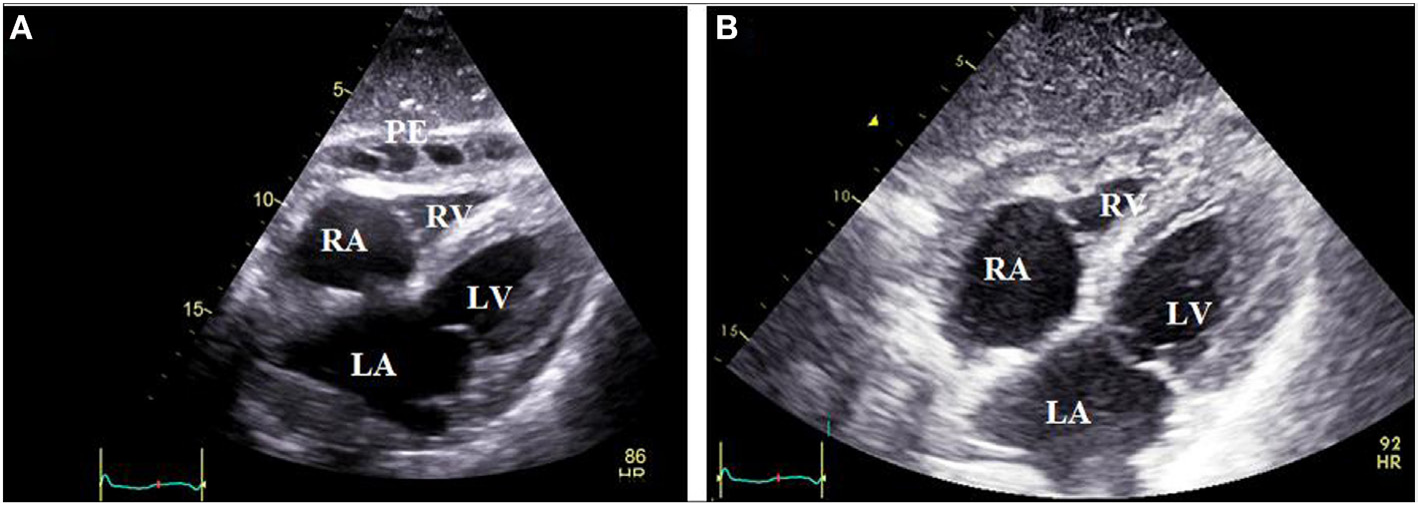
Subxiphoid echocardiographic view of a patient with colchicine-resistant steroid dependent idiopathic recurrent pericarditis. Panel (A) depicts pericardial effusion mainly in the anterior pericardial space with thick adhesions between visceral and parietal pericardium, which developed during the steroid tapering process. Panel (B) reveals absence of effusion in the same patient 1 month after switching from steroids to anakinra therapy (100 mg subcutaneously daily). PE, pericardial effusion; LA, left atrium; RA, right atrium; LV, left ventricle; RV, right ventricle.
